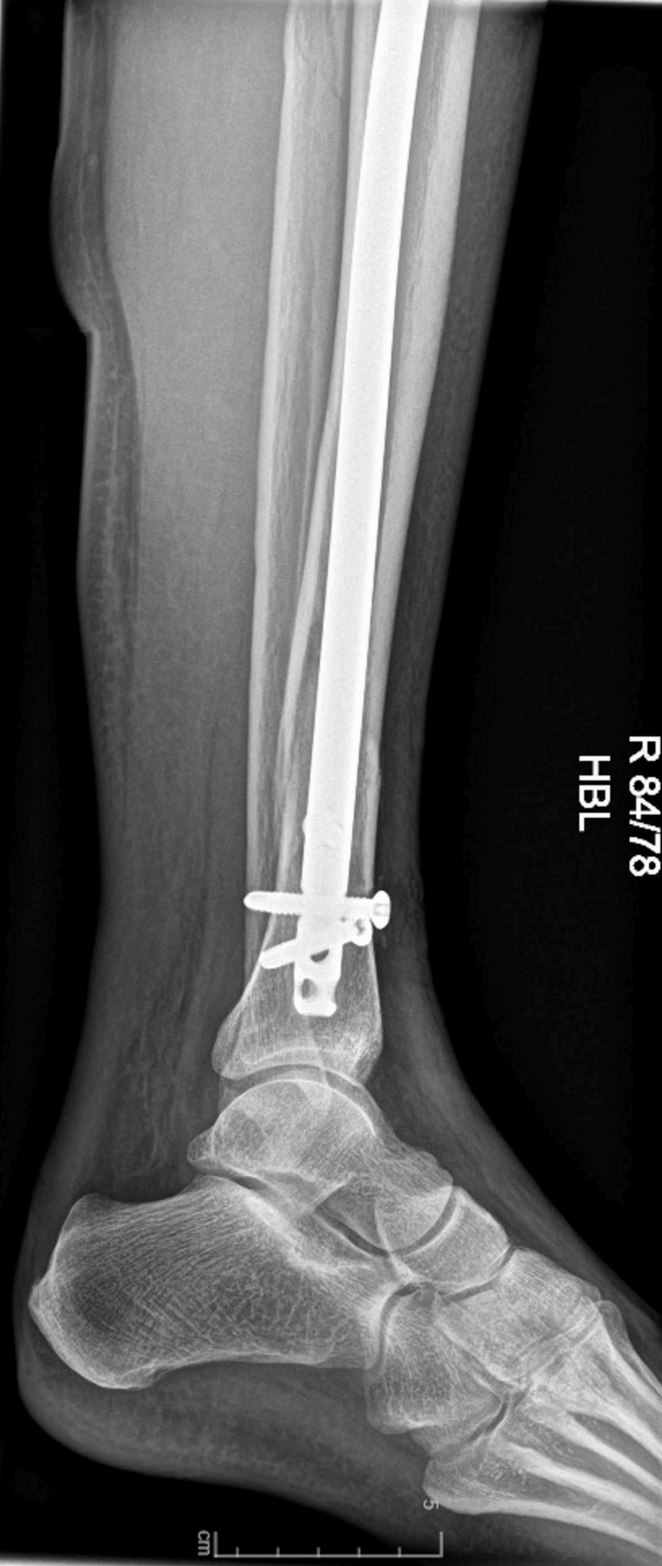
A postoperative X-ray from the lateral view.
